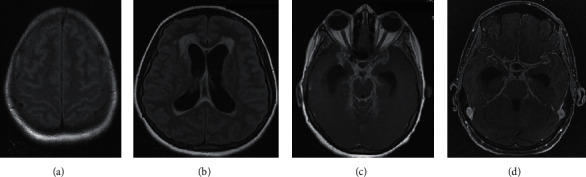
Nuclear magnetic resonance in case 2. (a) Hypersignal in the cerebral turns, bilaterally high convexity in T2 FLAIR. (b) Moderate dilated supratentorial ventricular system, associated with periventricular white matter hypersignal, probable CSF transudation on T2 FLAIR. (c)-(d) After administration of venous contrast, intense diffuse meningeal enhancement markedly in the regions of the cisterns of the base, axial T1, and Vol T1 3D, respectively.
